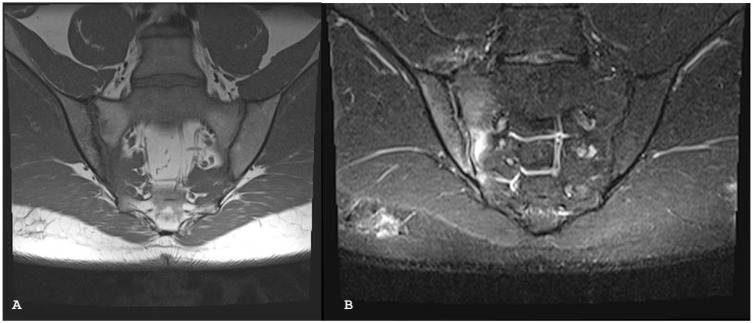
Oblique coronal T1-weighted magnetic resonance imaging (A) and oblique coronal short tau inversion recovery (B) sequences show periarticular bone marrow edema involving the right sacroiliac joint associated with multiple subchondral erosions, the confluence of which has resulted in the pseudo-widened appearance of the right sacroiliac joint. Capsulitis can be visible anteriorly (B). Note that the erosions are more on the iliac, rather than the sacral, side of the joint because the articular cartilage overlying the iliac side is half as thin as that on the sacral side.
